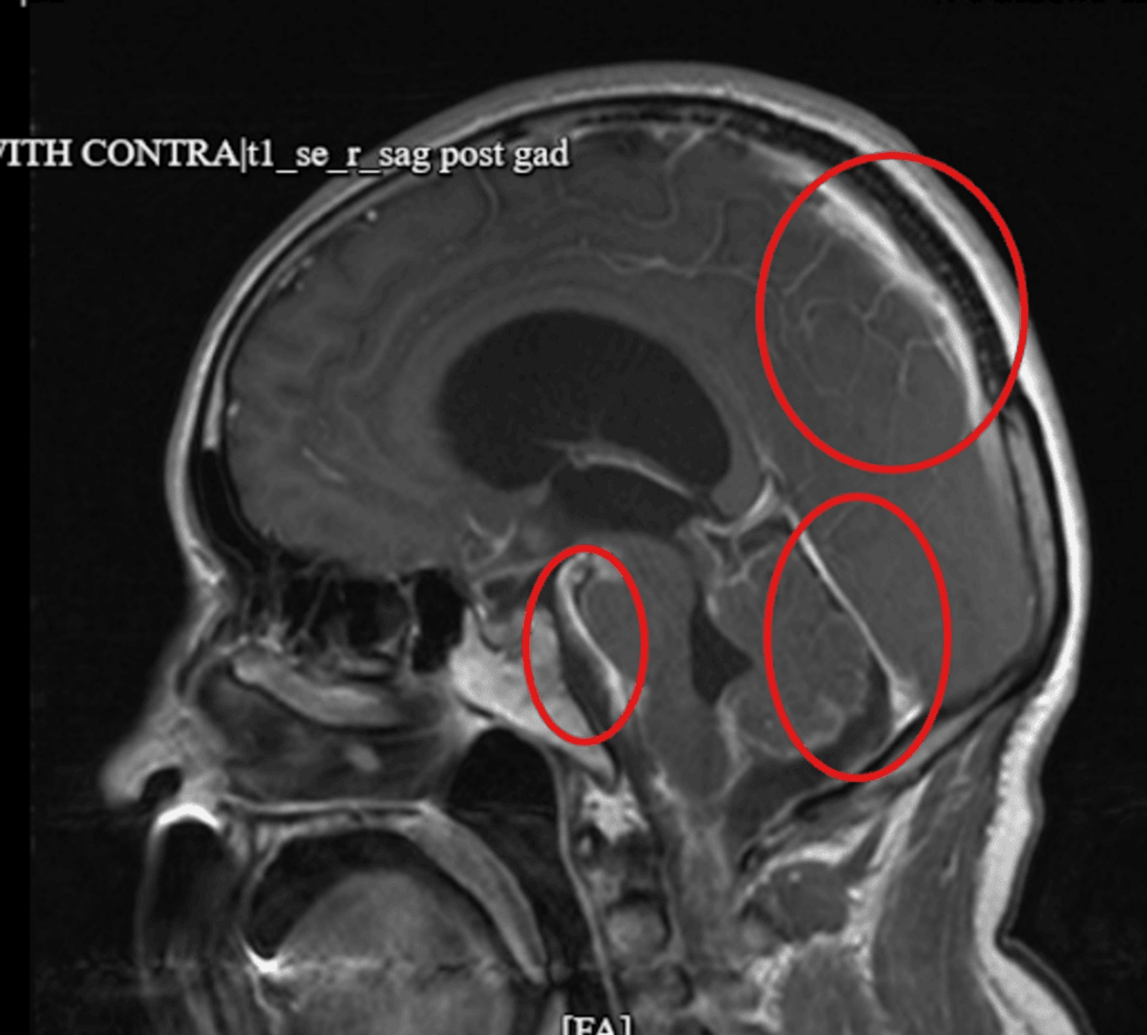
Brain magnetic resonance imaging demonstrating communicating hydrocephalus with transependymal cerebrospinal fluid flow and multiple enhancing lesions surrounding the brainstem and basilar cisterns with diffuse subarachnoid enhancement consistent with a leptomeningeal process
